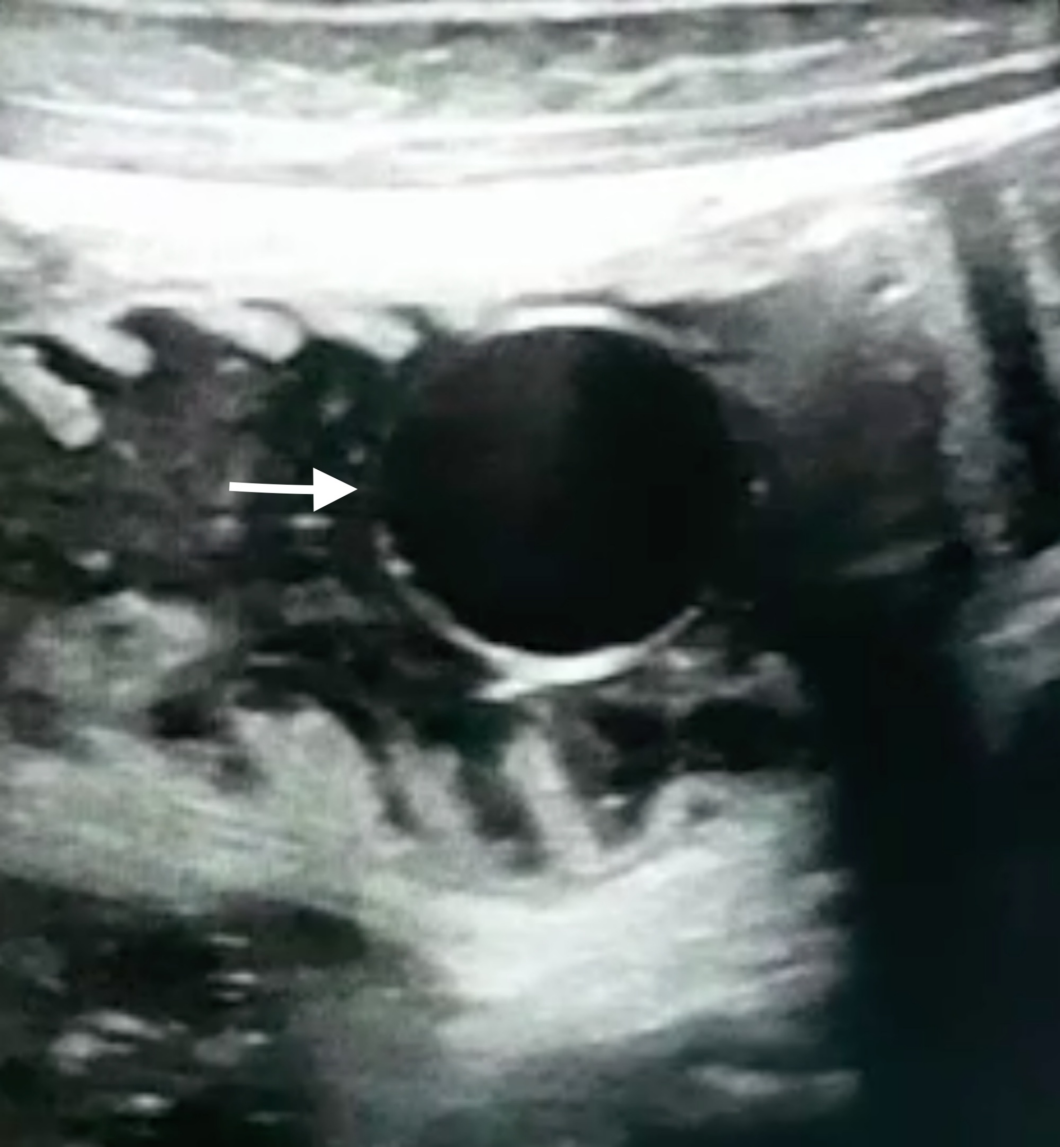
Sonographic image showing intraluminal cyst within a jejunal loop. White arrow depicts the cyst.
